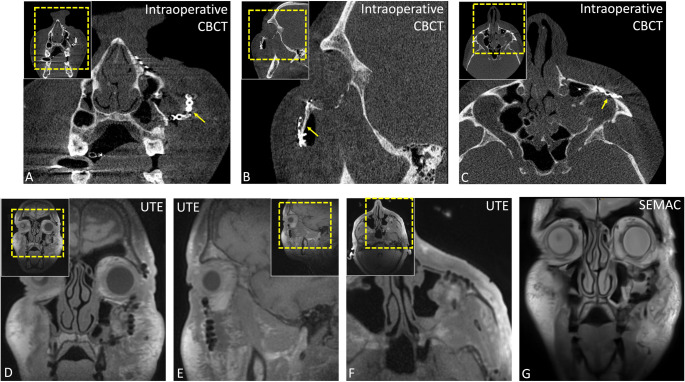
Multiple fractures of the left midface. Open reduction and internal fixation (ORIF) of the left zygomatic fracture, including the zygomatic arch attachment (arrow). Intraoperative imaging was performed using X-ray-based C-arm CBCT (A-C), and radiation-free, CT-like depiction was obtained with MRI using UTE (D-F), and visualization with the SEMAC (G) sequence. CBCT: Cone-beam computed tomography; UTE: 3D ultrashort echo time; SEMAC: Slice-encoding for metal artifact correction sequence; DESS: 3D double-echo steady-state
